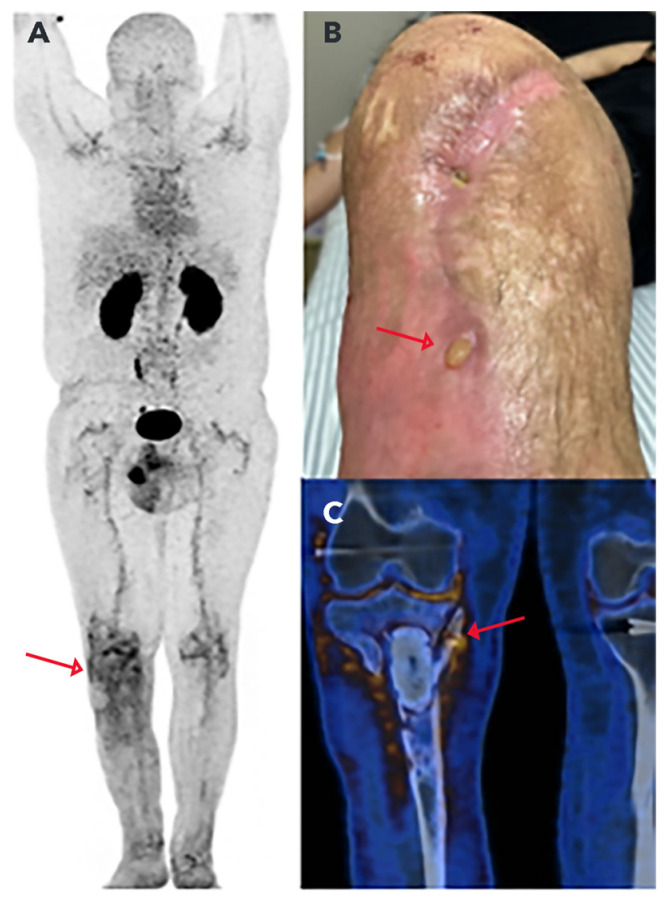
(A) Biodistribution of [^68^Ga]Ga-DOTA-UBI_29-41_ in the whole-body PET/CT image in maximum intensity projection (MIP). The arrow indicates the increased uptake of the radiopharmaceutical in the right knee. (B) The arrow indicates the lesion with purulent secretion in the proximal portion of the right leg. (C) Fusion of the PET/CT images, where the arrow highlights the uptake of the radiopharmaceutical in the right proximal tibia.
